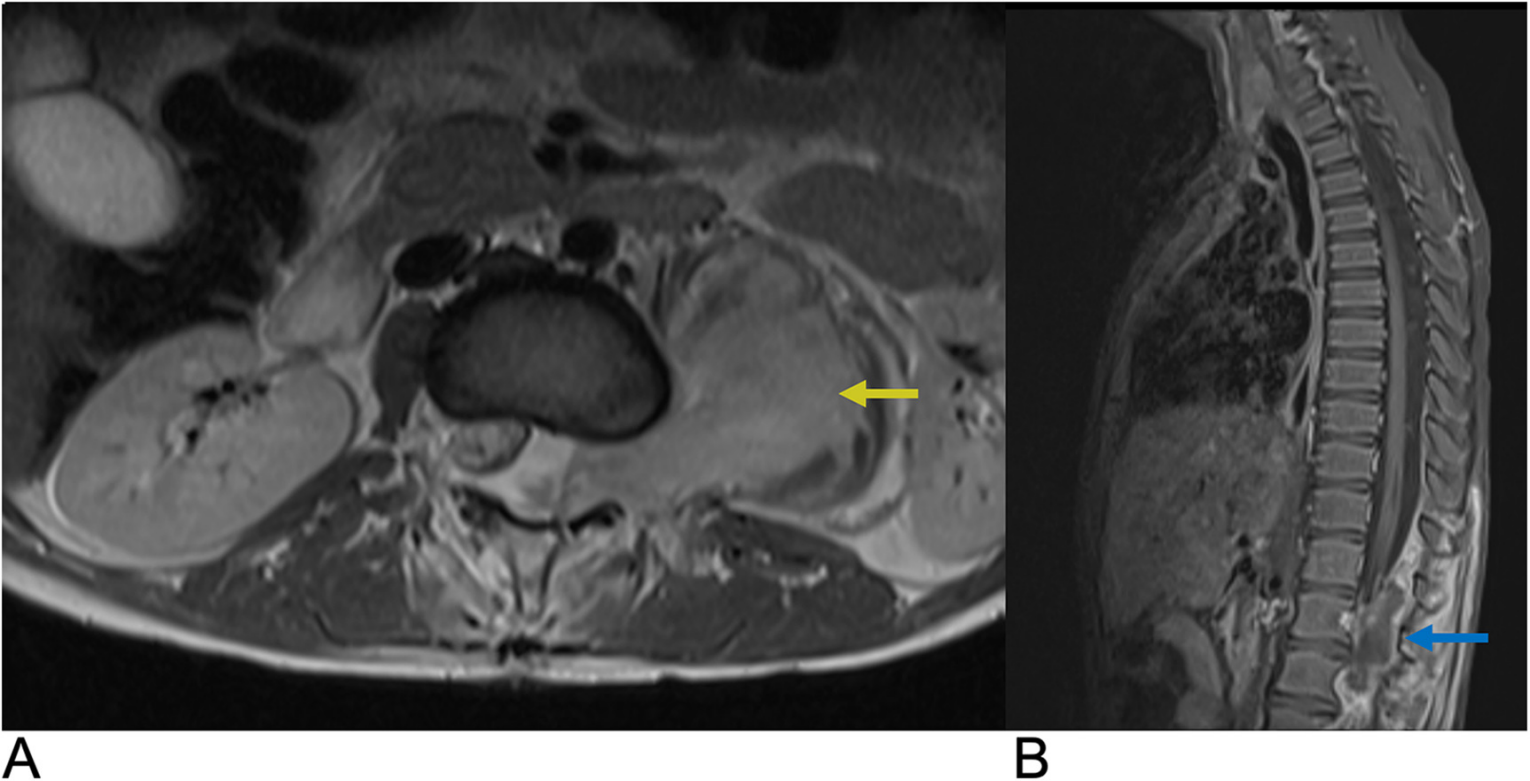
Epidural tumor. Axial T1 + C (A) and Sagittal T1 + C (B) MRI demonstrating an enhancing epidural malignant lesion (blue arrow) extending laterally through the neural foramen with infiltration of the left psoas muscle (yellow arrow) and associated spinal cord compression.
